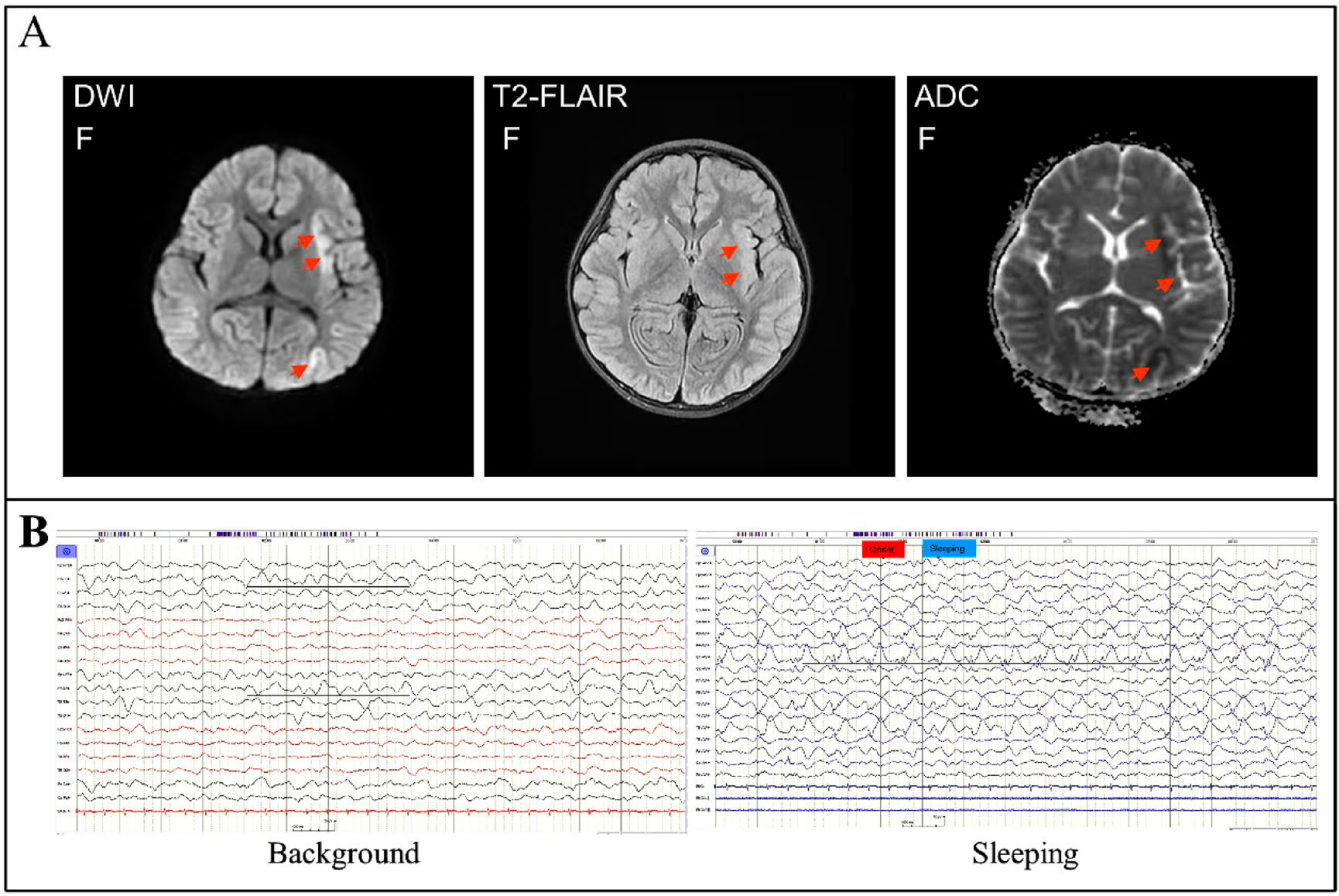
Brain imaging and Electroencephalogram (EEG). Panel A: Magnetic resonance imaging (MRI) of the brain performed on day 6 of the illness showed obvious abnormal signals on DWI (abnormally hyperintensity in the left insula and parietal lobe), ADC (abnormally hypointensity in the left insula and parietal lobe), and T2-FLAIR images (slightly hyperintensity in the left insula lobe) (marked with an arrow). Panel B: EEG on day 6 of the illness presented the relative enhanced θ and δ activities with the continuous asymmetrical low waves manifested spike, sharp, or slow wave complex (marked with a black line).
